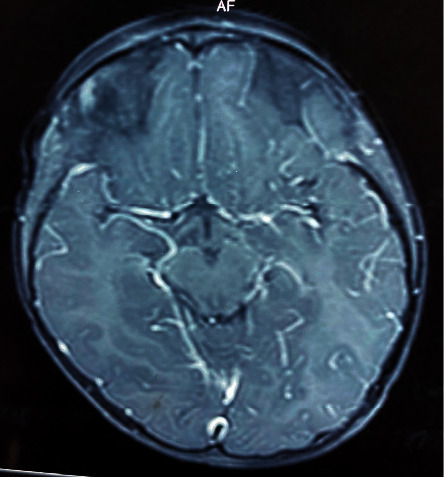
MRI brain performed three months after treatment, showing marked improvement in signal intensity changes in the midbrain.
